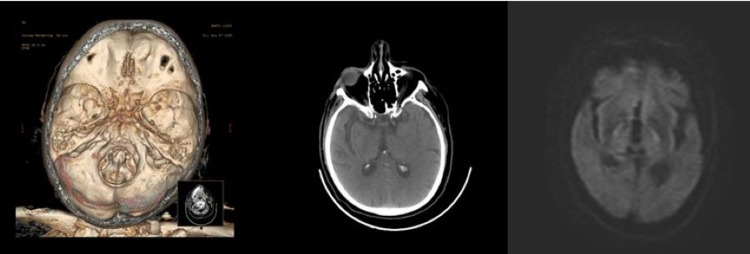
Volume-rendered image does not demonstrate any vessel occlusion. Focal abnormalities are not observed in the brain parenchyma at CT scan and DW images. DW: diffusion-weighted
